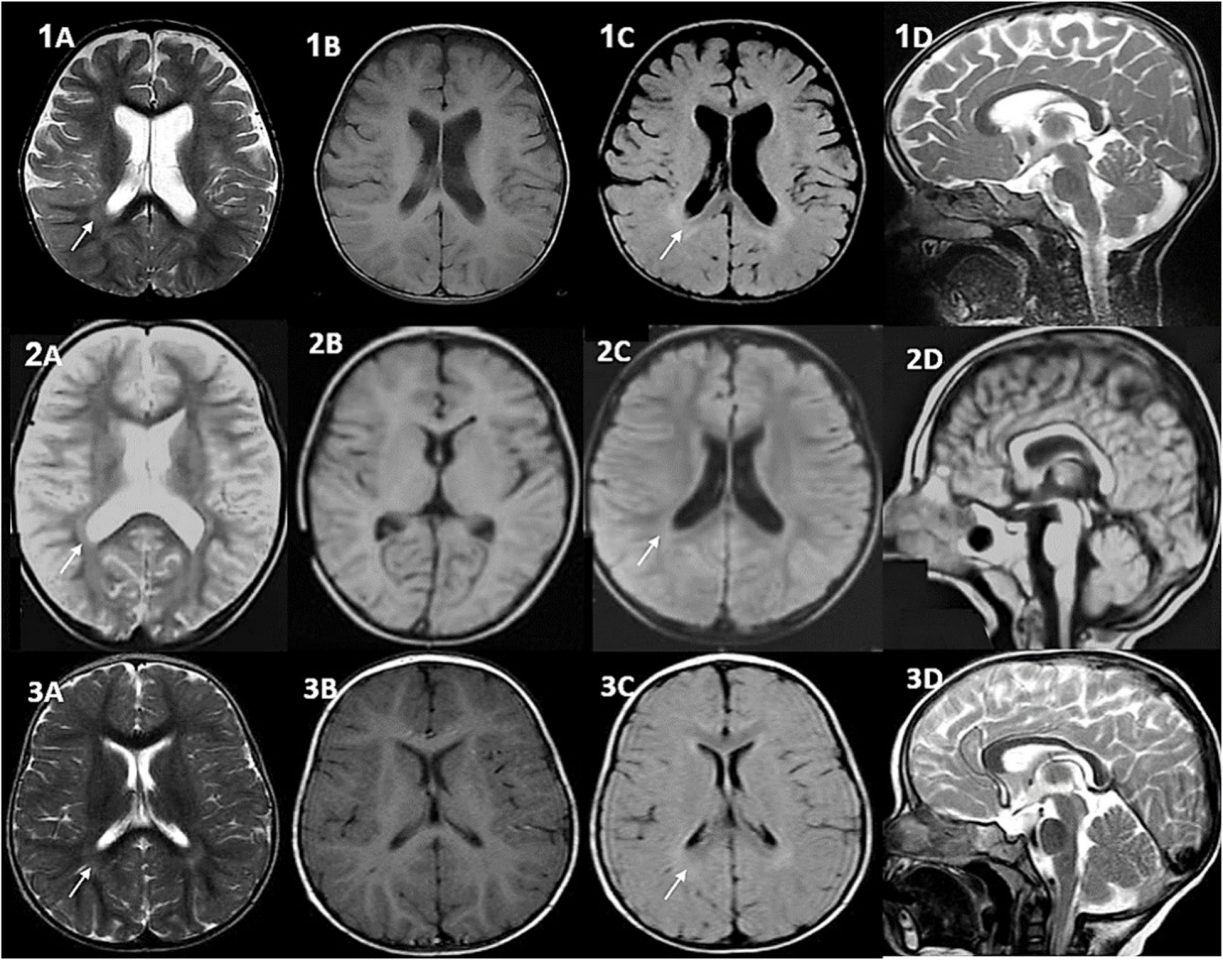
Brain MRI sequences of P1 (1A–D), P2 (2A–D), and P3 (3A–D) without Gad at the age of 18 months. Posterior periventricular and deep white matter signal changes in axial T2-weighted and FLAIR images (1A, 1C–2A, 2C–3A, 3C white arrows), deepening of the Sylvain fissures (1A), mild supratentorial atrophy and exvacue ventriculomegaly (IA, 1C, 2A), near-normal appearance of axial and sagittal T1-weighted imaged (1B–2B–3B, 2D), and thinning of the corpus callosum (1D, 3D) were the most prominent imaging findings. In addition, delayed myelination in subcortical white matter is seen at the age of 18 months (3A)
